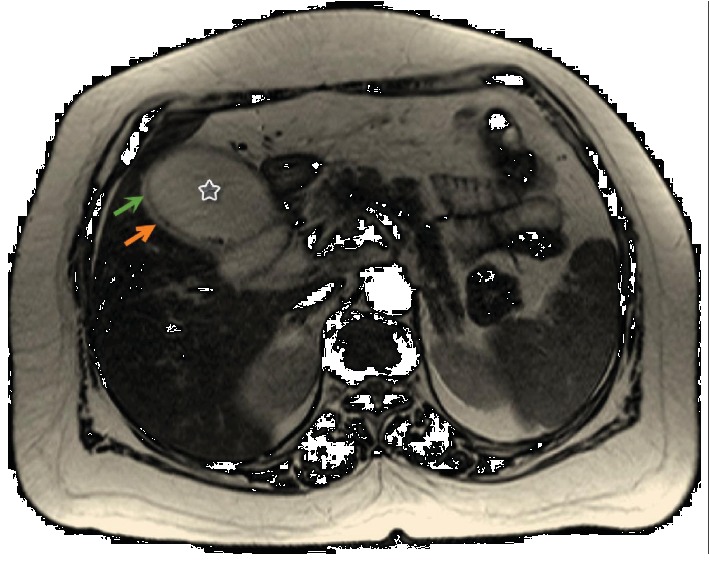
Magnetic resonance imaging (MRI) of the abdomen. Axial slice obtained through the distended GB (star) shows the thin gall bladder wall (green arrow) and a thin rim of pericholecystic fluid (orange arrow).
